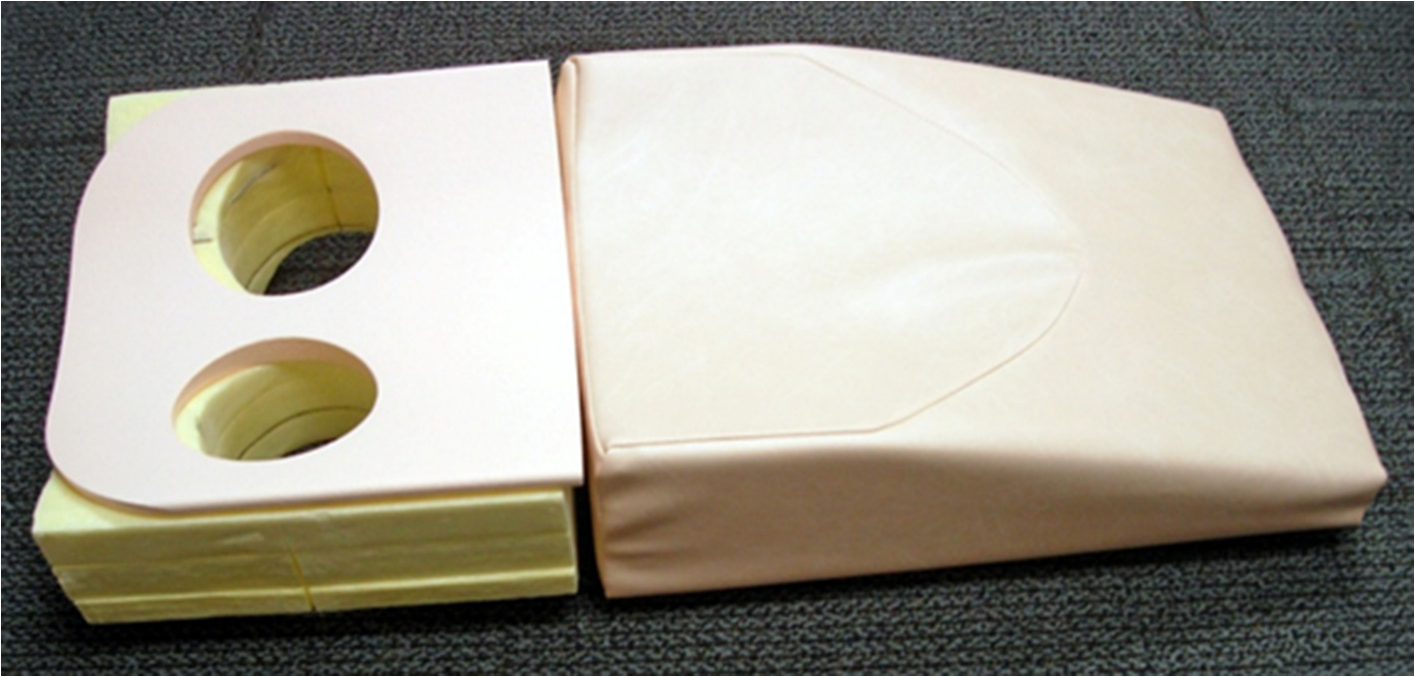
Our own support for prone breast PET/CT images. It allows for the breasts to lie pendant during the scanning procedure, therefore greatly enhancing the ability to perform longitudinal registration. Typical breast PET/CT is performed in the supine position which results in less reproducible patient positioning between scan sessions.
